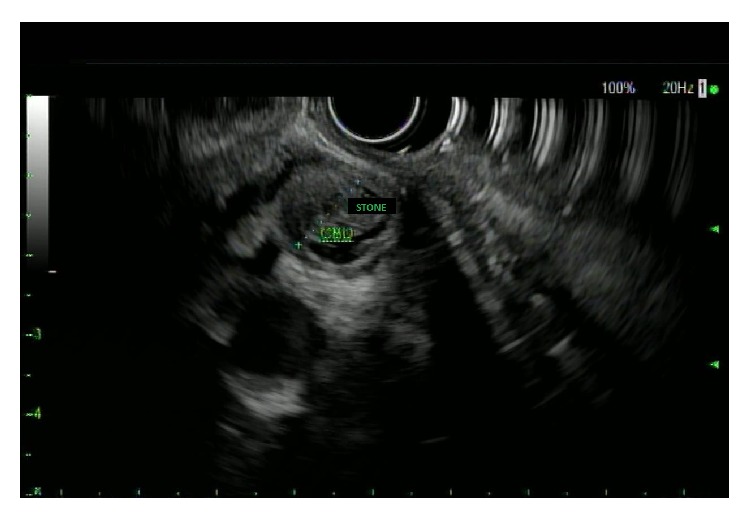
Upper endoscopic ultrasound.
